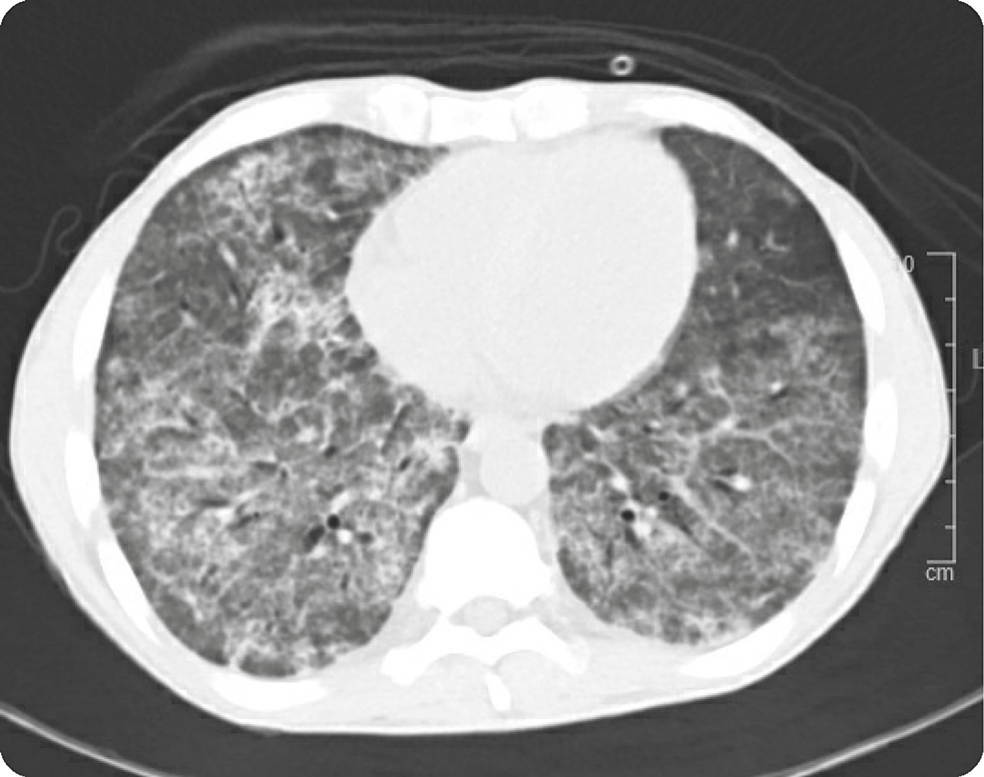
CT chest on day 8, improved nodularity is noticed.
